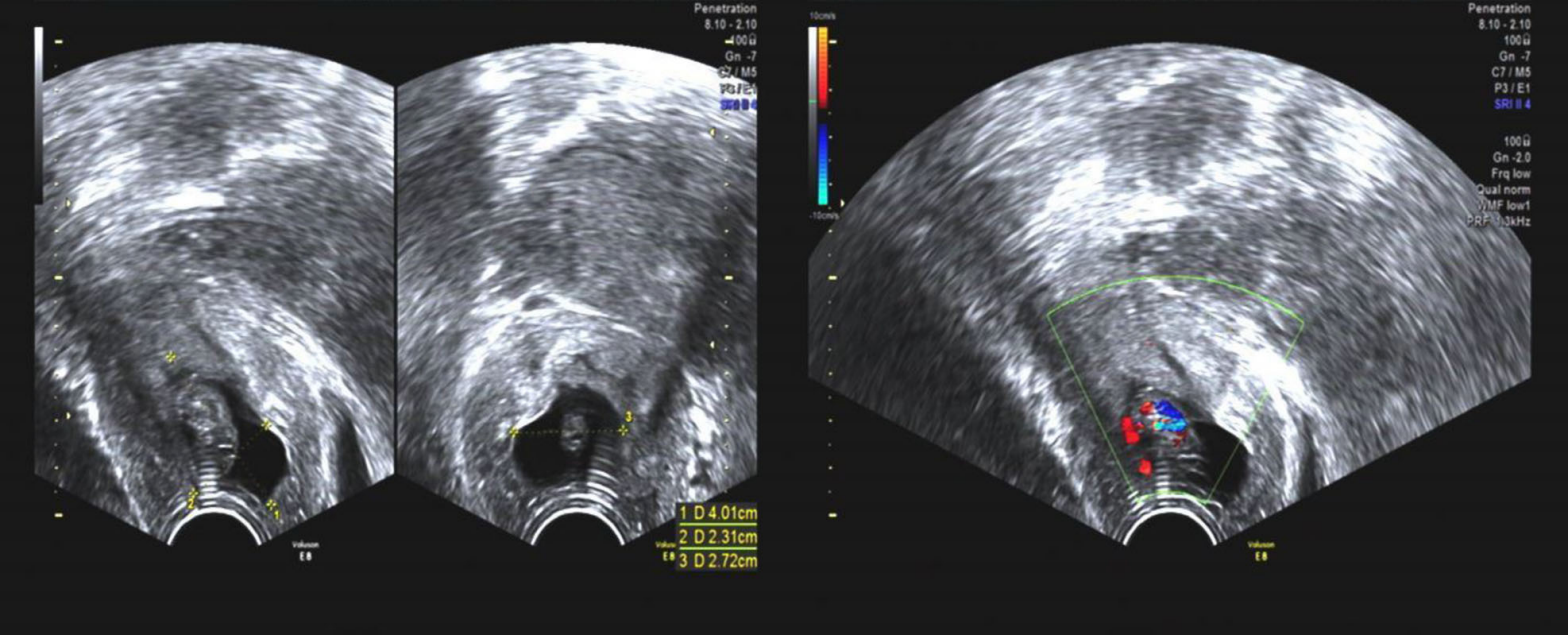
Case 1 ultrasound imaging of cervical pregnancy at the time of diagnosis
